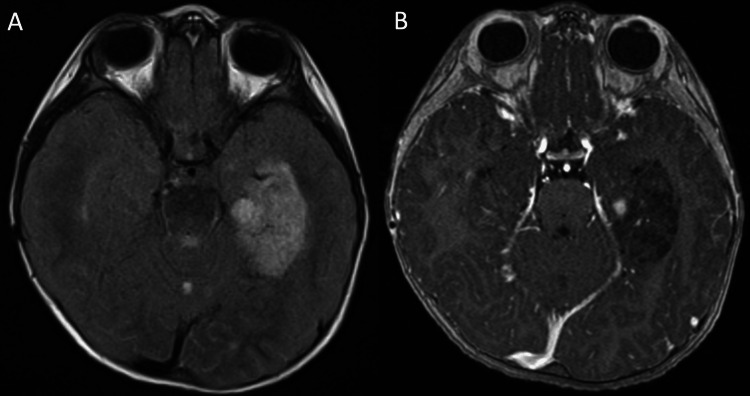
Axial MRI demonstrating left temporal and cerebellar vermis lesions prior to initial resection. (A) T2-FLAIR. (B) Contrast T1.
